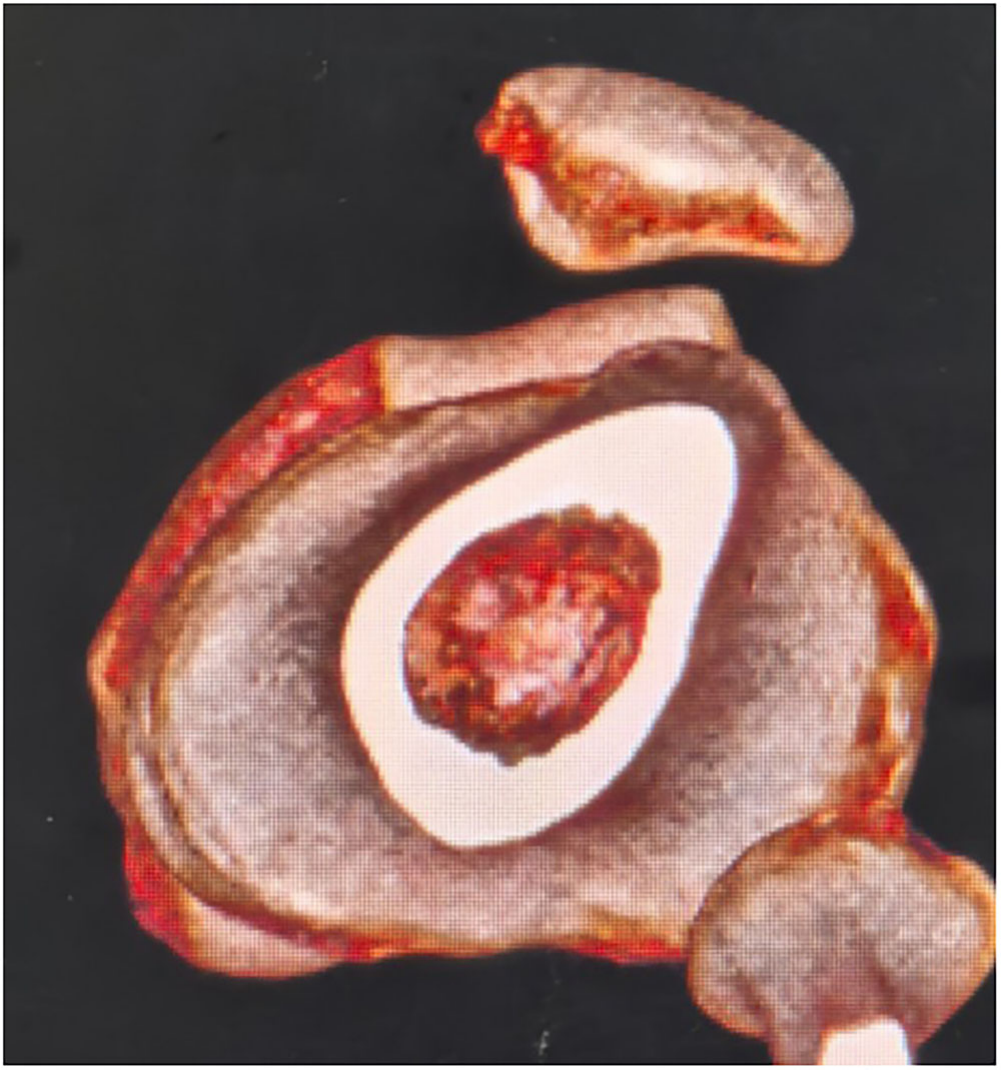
Preoperative 3D CT revealed patellar subluxation. 3D, three‐dimensional; CT, computed tomography.
